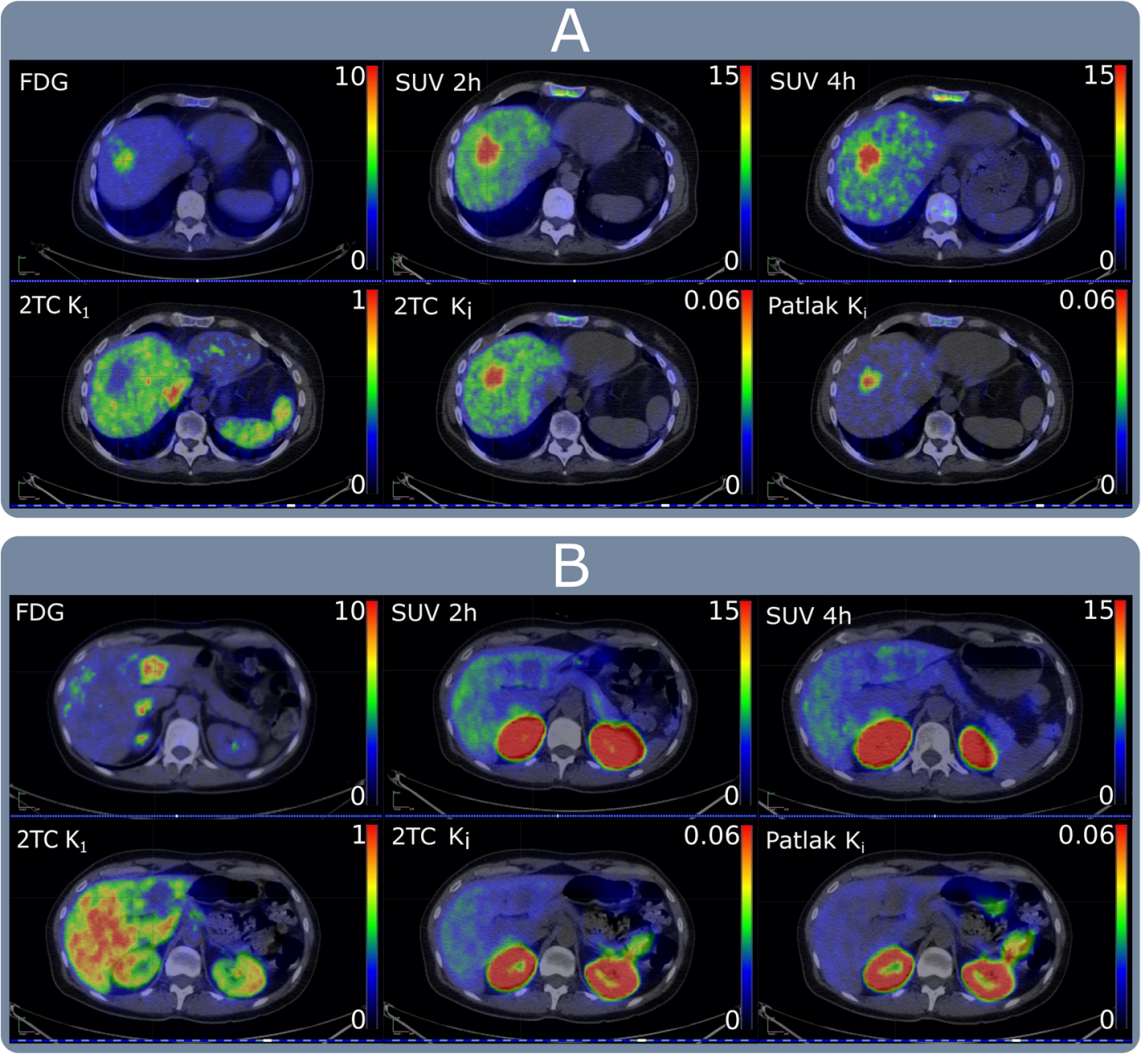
Positron emission tomography (PET) images. a In a patient with HER2-positive expression. b In a patient with HER2-negative expression. ^18^F-FDG PET images to the left followed by SUV static image taken at 2 h time point after ^68^Ga-ABY-025 injection. 2TC *K*_*i*_, Patlak *K*_*i*_, and (2TC) *K*_1_ are parametric images created from the ^68^Ga-ABY-025 dynamic PET study (*K*_1_, transfer rate constant from plasma to the tissue compartment; *K*_*i*_, influx rate of the tracer into irreversible compartment)
